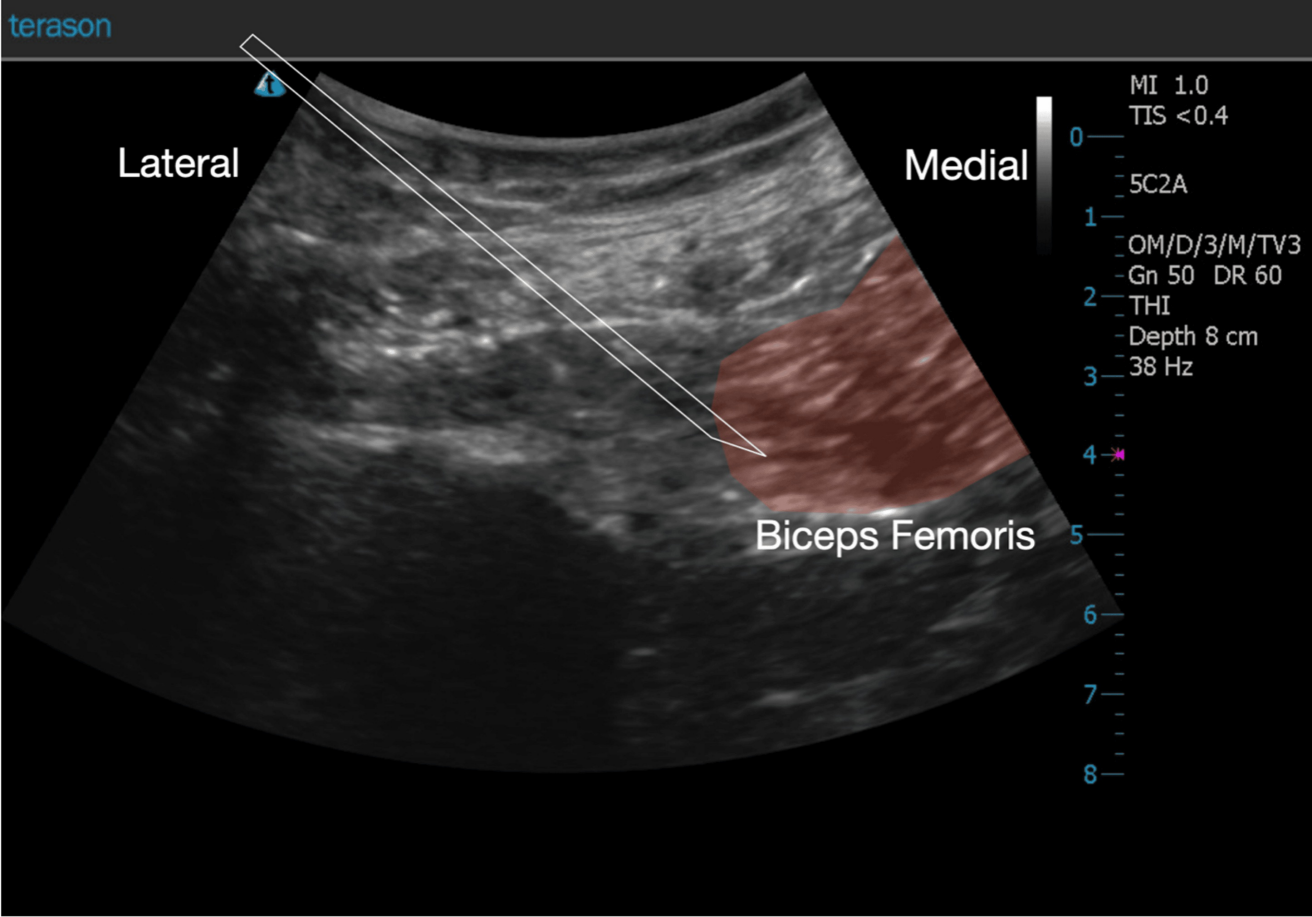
This colored sonogram highlights the short-axis view of the avulsed biceps femoris muscle. This injured muscle is highlighted in red. Note the irregular streaking throughout the muscle. This is relatively inconsistent when compared to non-injured muscle grain appearance. The needle position is outlined to accentuate the trajectory and demonstrate resting position.
